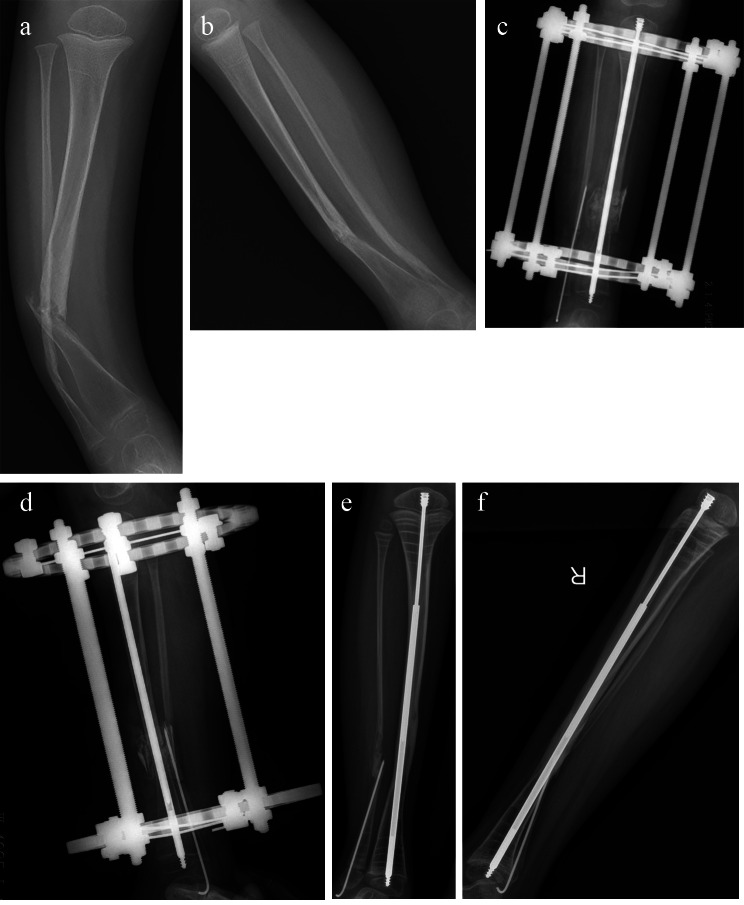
Female CPT children aged 3 years, A and B: Anteroposterior and lateral radiographs before operation; C, D: Three days after operation, X ray of tibia showed that the new type of children’s extensible intramedullary rod was in good position; E. F: Two years and three months after the operation, X ray of the tibia showed good healing at the tibial pseudarthrosis, no proximal tibial valgus and no intramedullary rod displacement
